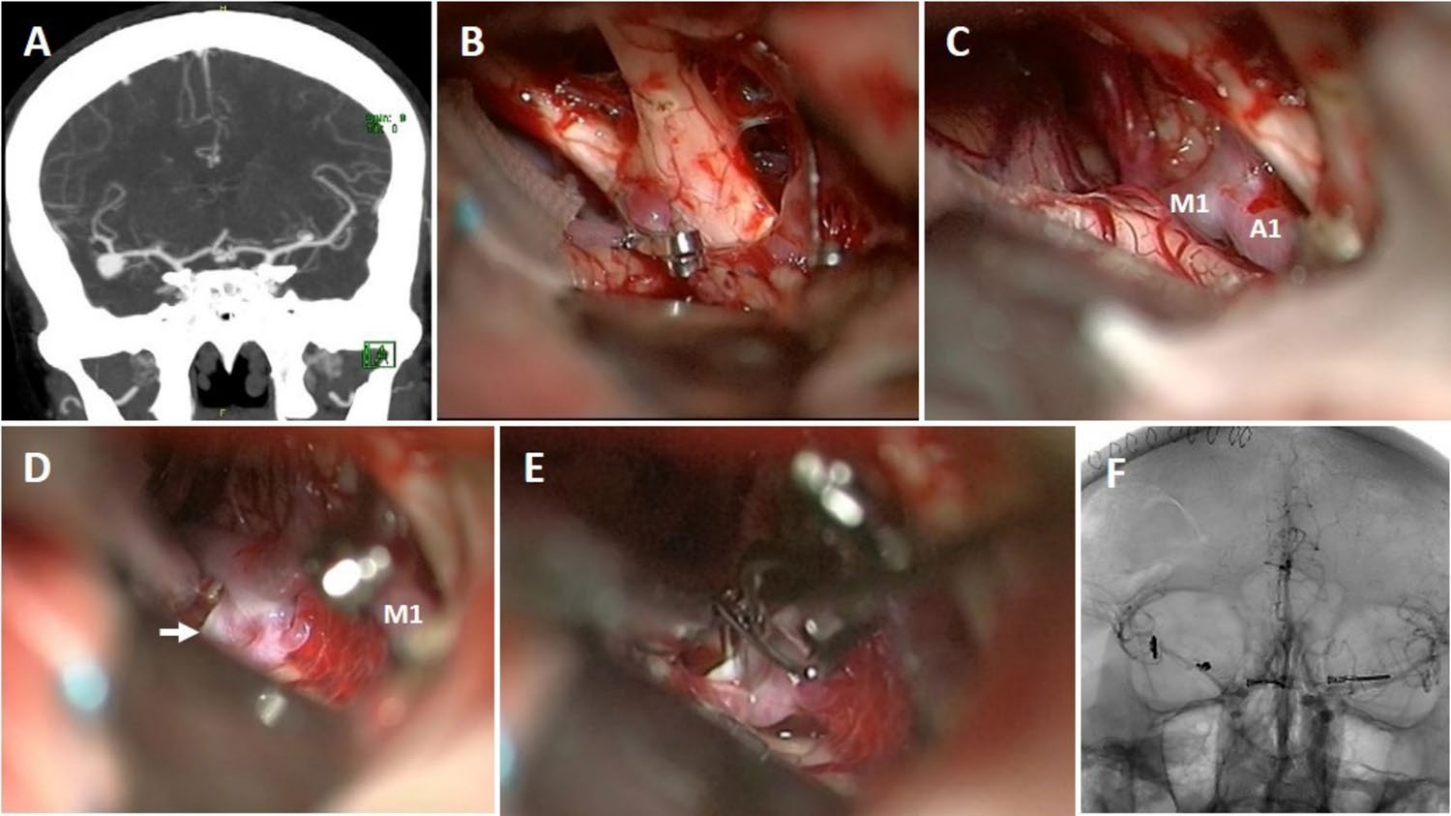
A Coronal section of CT-angiogram shows four aneurysms: right MCA bifurcation and M1 segment, ACoA and left M1 segment, B After two aneurysms in the right MCA were clipped, the ACoA aneurysm was explored and clipped with a small slightly curved clip, C The left ICA bifurcation was reached by following the left A1 segment, D the small aneurysm at the left M1-Anterior temporal artery junction was explored (arrow) using the temporary clip, E and clipped with a sideward clip, F control angiogram confirmed total occlusion of all four aneurysms
